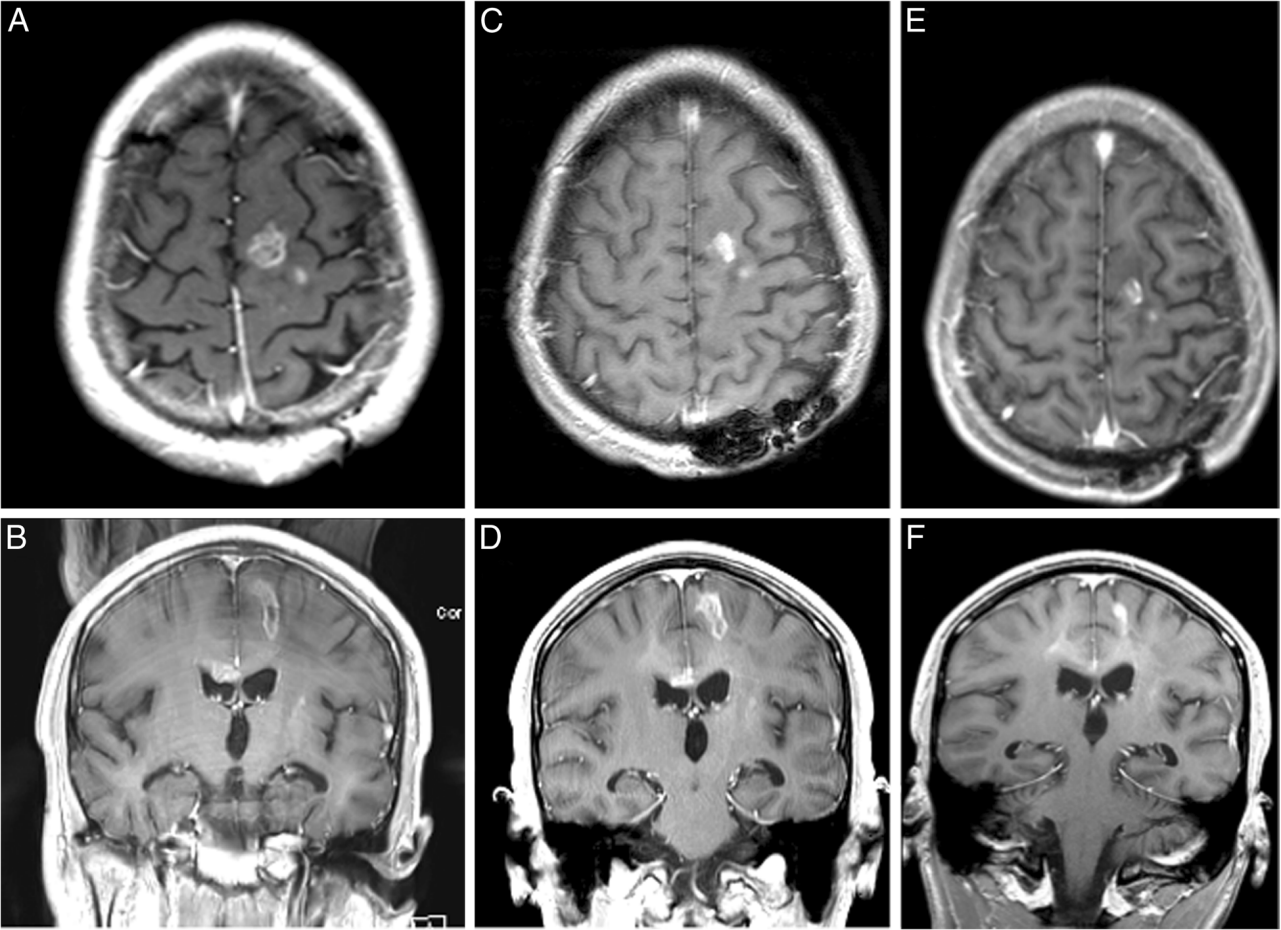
Partial response in-patient following vaccination. Axial and coronal MR images of a 17-year-old male with recurrent glioblastoma multiforme (patient 5) showing a partial radiographic response. Images show his scans at initiation of therapy (A, B), at week 8 (C, D) and week 20 (E, F).
